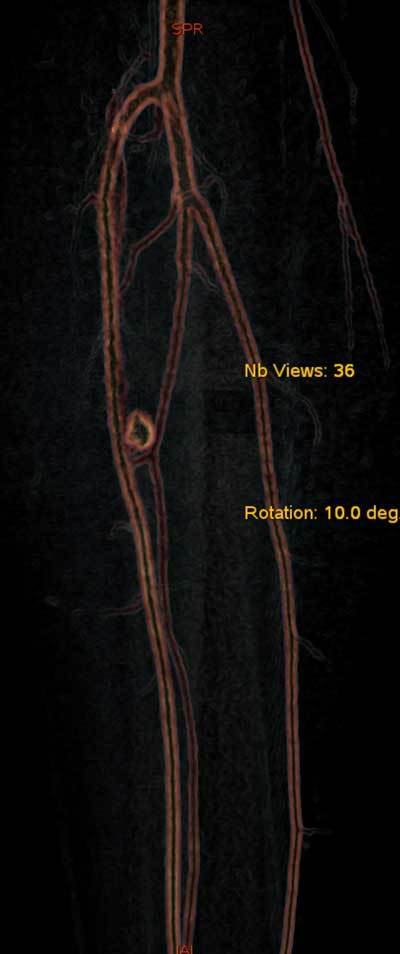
Multiplanar volume-rendering reformation CTA: pseudoaneurysm of the anterior tibial artery.
